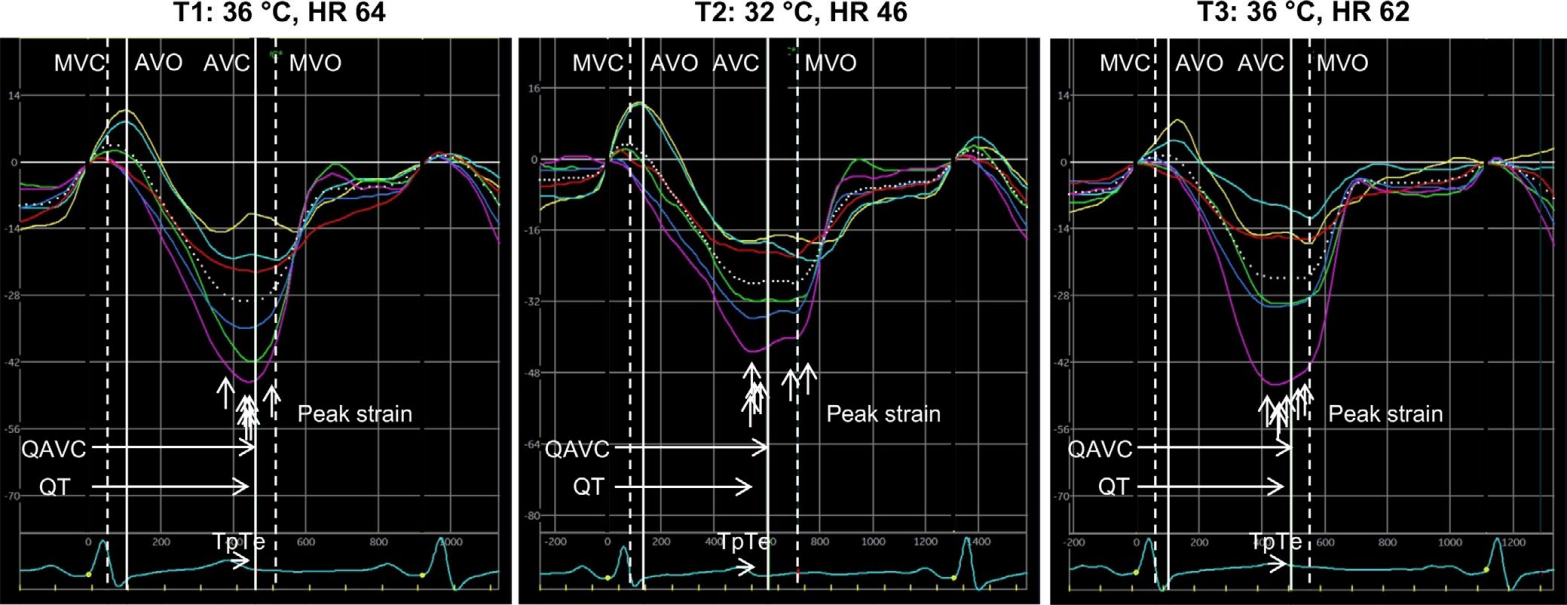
Longitudinal strain by speckle tracking echocardiography in apical long axis view at baseline: T1:36°C, at moderate hypothermia: T2:32°C and after rewarming: T3:36°C, at spontaneous heart rate (HR). White lines indicate aortic valve opening (AVO) and closing (AVC), and white dotted lines mitral valve opening (MVO) and closing (MVC). QAVC, QT‐interval and TpTe are marked. Electromechanical window is represented by the difference QAVC‐QT, dispersion of repolarization by the inter‐individual variance in TpTe, and mechanical dispersion as variation in time to peak strain, marked with white vertical arrows. Electromechanical window positivity is increased, while dispersion of repolarization and mechanical dispersion are unchanged at moderate hypothermia. Electromechanical window is returned to baseline value after rewarming
